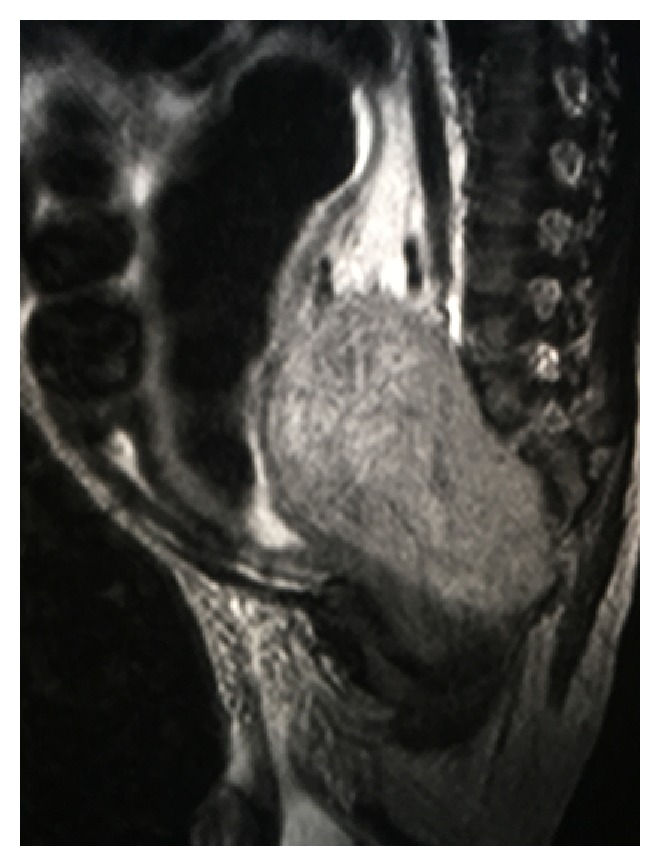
MRI image showing mass extending to sacral vertebras.
